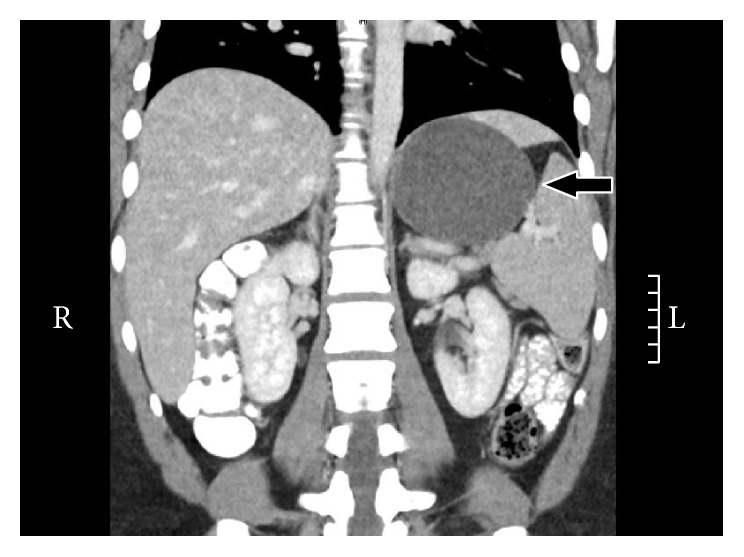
Computed tomography of intra-abdominal cysts. Arrow points to bronchogenic cyst arising from the stomach wall (coronal).
